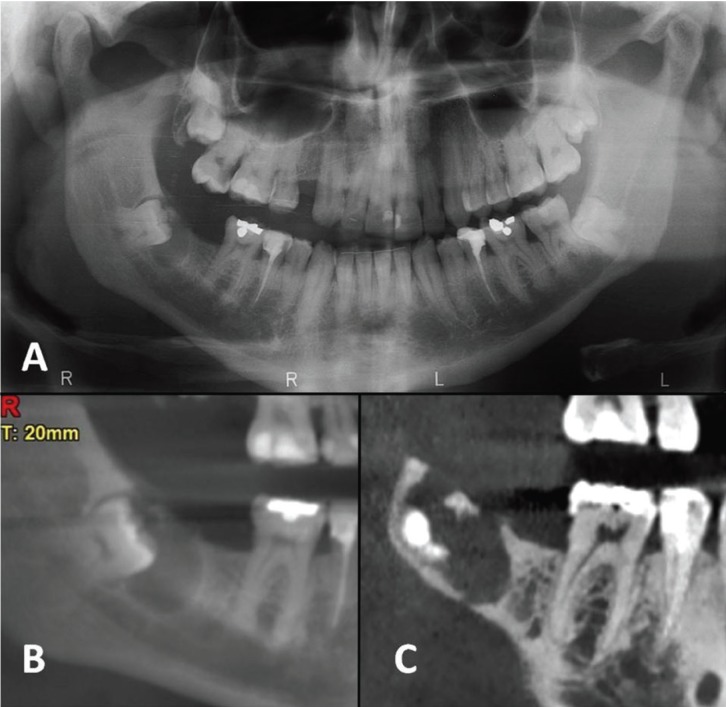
A) Ortopantomography shows a radiolucent pericoronal lesion associated to the right lower third molar with a radiopaque material inside. B) Panoramic slice from computed tomography (CT). Note the distal bone sclerosis and calcifications. C) Sagittal slice from CT shows the alveolar ridge resorption.
